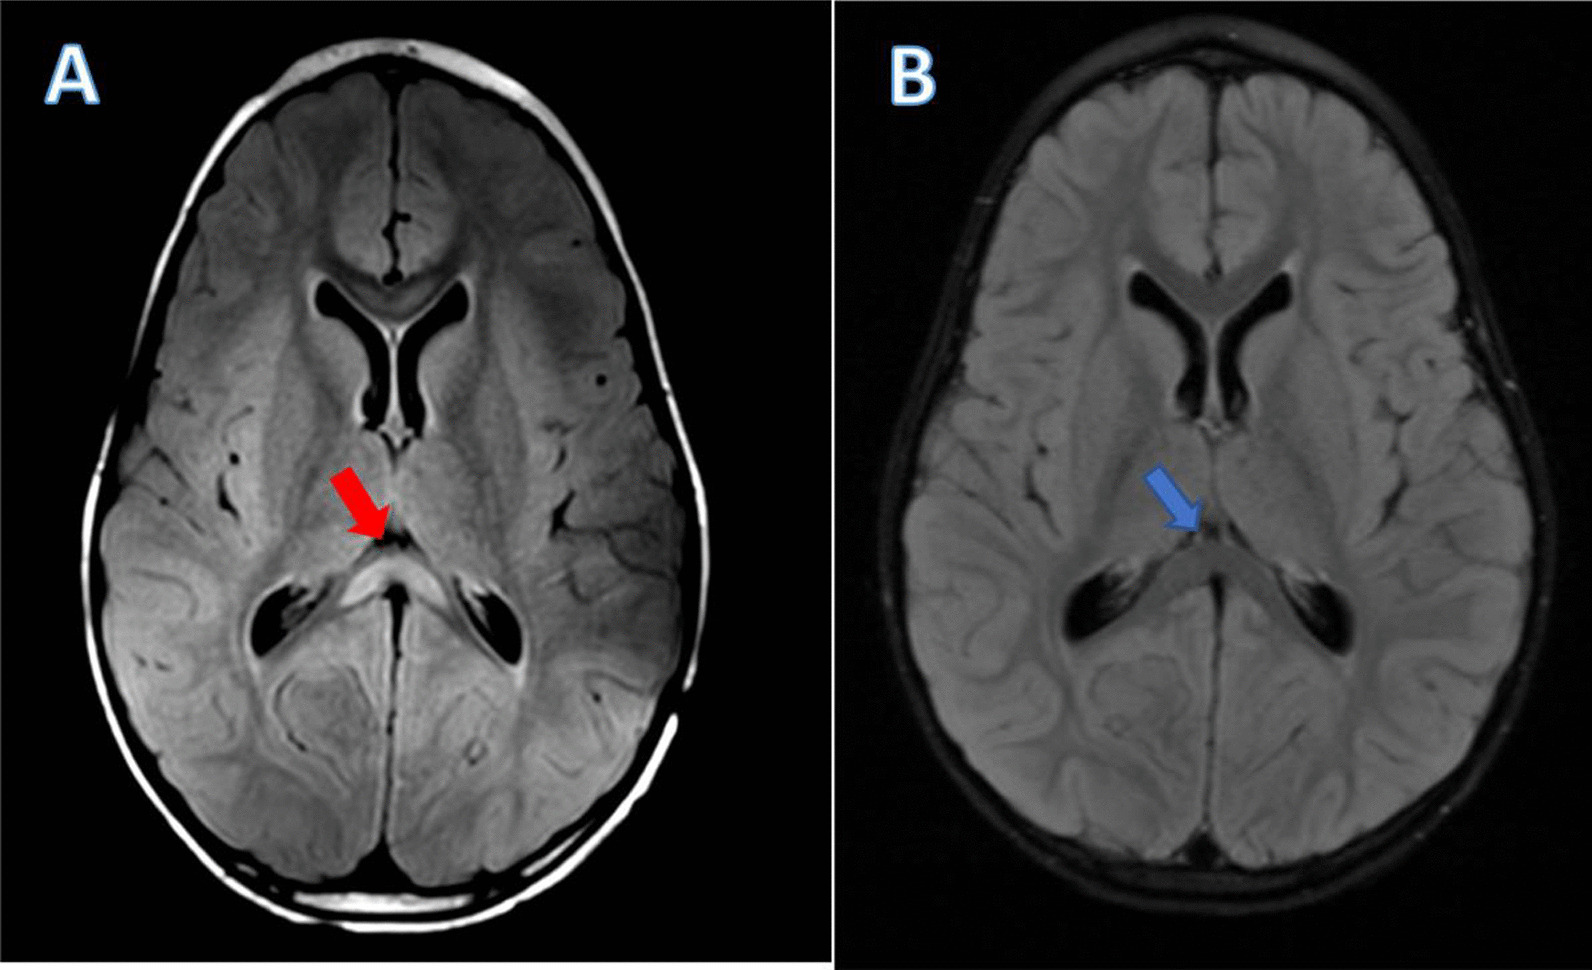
Neuroimaging demonstrating splenium of corpus callosum hyperintensity. Brain magnetic resonance imaging T2 fluid-attenuated inversion recovery sequence demonstrating A hyperintensity involving the splenium of the corpus callosum (red arrow), aka the “Boomerang Sign” [16], with B resolution of hyperintense signal on repeat imaging after 5 days (blue arrow) since initial imaging, and 2 days after pulse-dose intravenous steroids.
